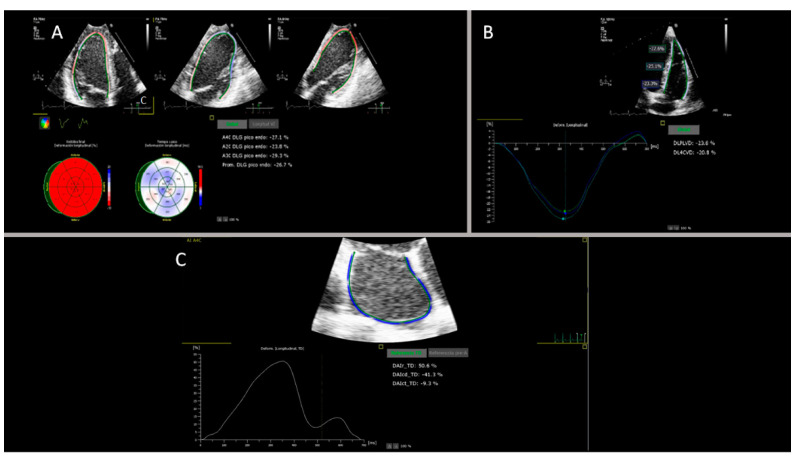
Panel (A): Output of the left ventricular longitudinal strain analyses. The upper part shows, from left to right, the endocardial tracking of chambers four, two and three; the bottom left part shows the bull’s eye with the strain values (%) and the time to peak longitudinal strain (ms) for the 18 segments. On the bottom right part, the LVGLS results for chambers four, two and three and the average LVGLS (%) can be found. Panel (B): Output of the right ventricular longitudinal strain analyses. The upper part shows the endocardial tracing in the four apical chambers: basal, medial, apical and RVFWLS: (%). The bottom left part shows the deformation curves versus the time for each segment (coloured curves). On the right, the results of the average RVFWLS and the average RV4CLS (%) are found. Panel (C): Output of the left atrium longitudinal strain analyses. At the top is the apical four-chamber endocardial tracing; at the bottom left is the strain versus time curve, and at the bottom right, the GLS results for each of the phases of the atrium (reservoir, conduit and contraction) (%) can be found.
